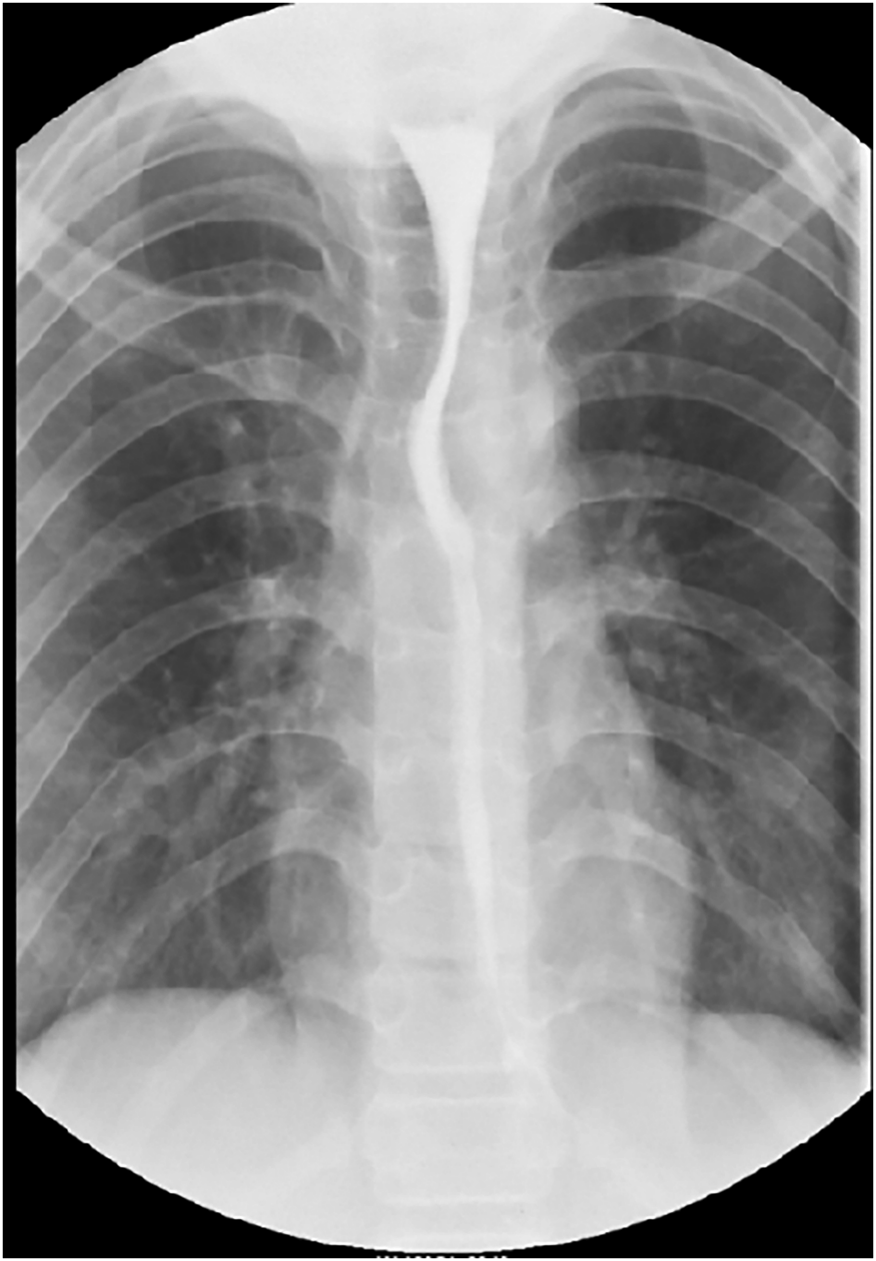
Upper gastrointestinal radiography showed diffusing contraction of the lower esophagus.
